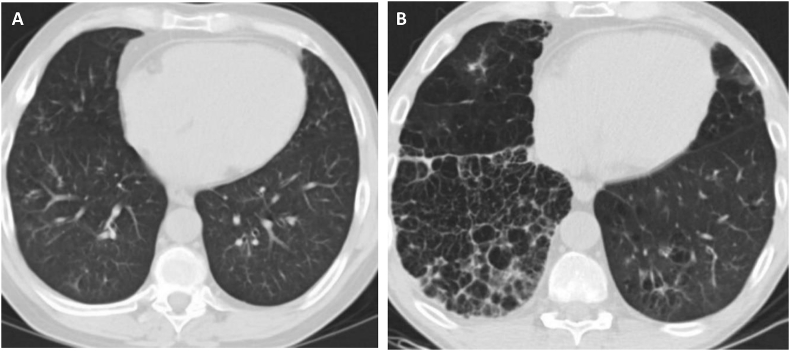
Chest CT scan with A) normal lung parenchyma one year following bilateral lung transplantation and B) bibasilar predominant emphysema (right greater than left), with pleural inflammation associated with chest tube placement, two years post-transplant CT, computed tomography.
